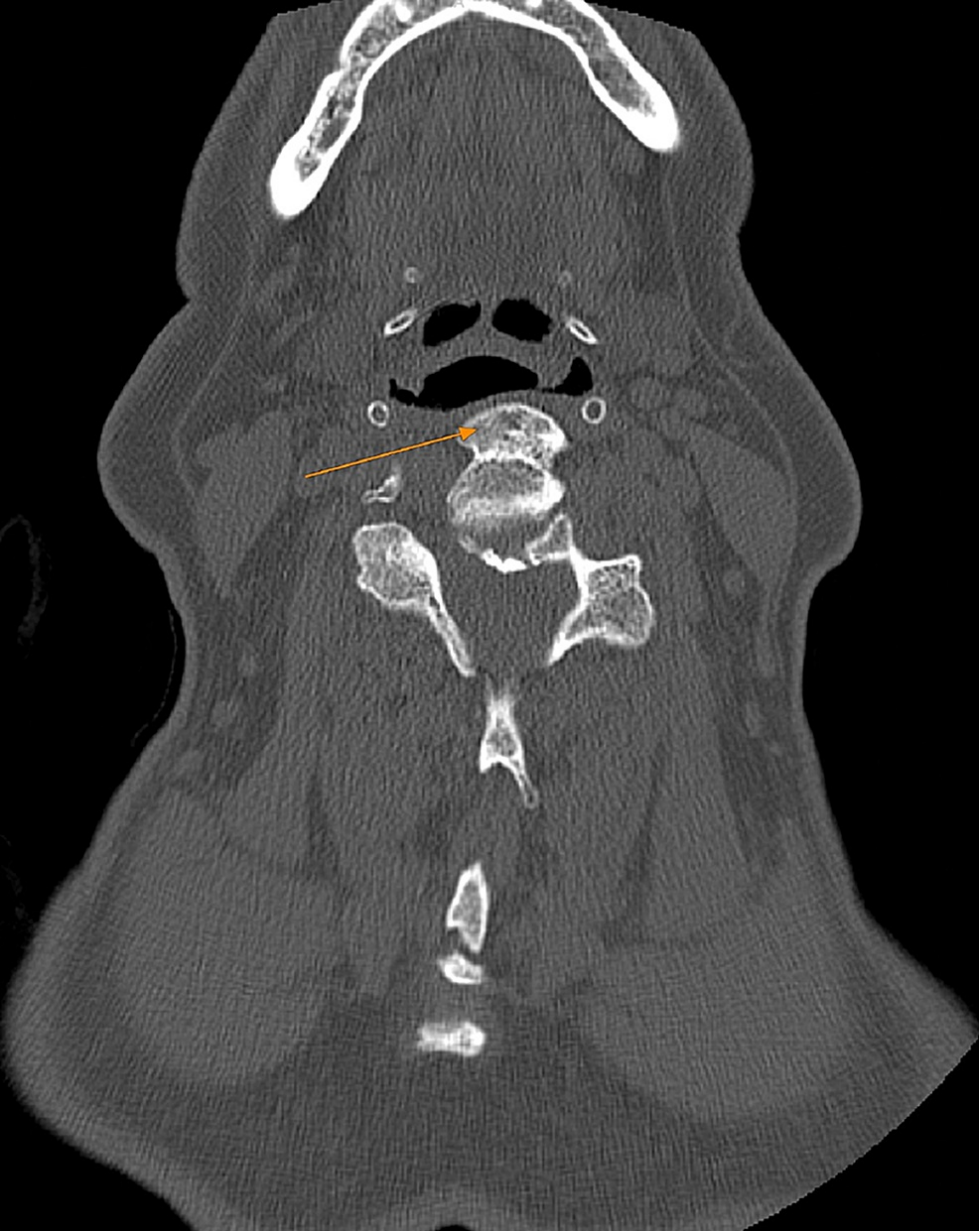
Transverse computed tomography demonstrating flattened anterior osteophytes which cause invasive phenomena and compress the upper airway. The arrow pointing to the osteophytes at the C3-C4 vertebral level.
